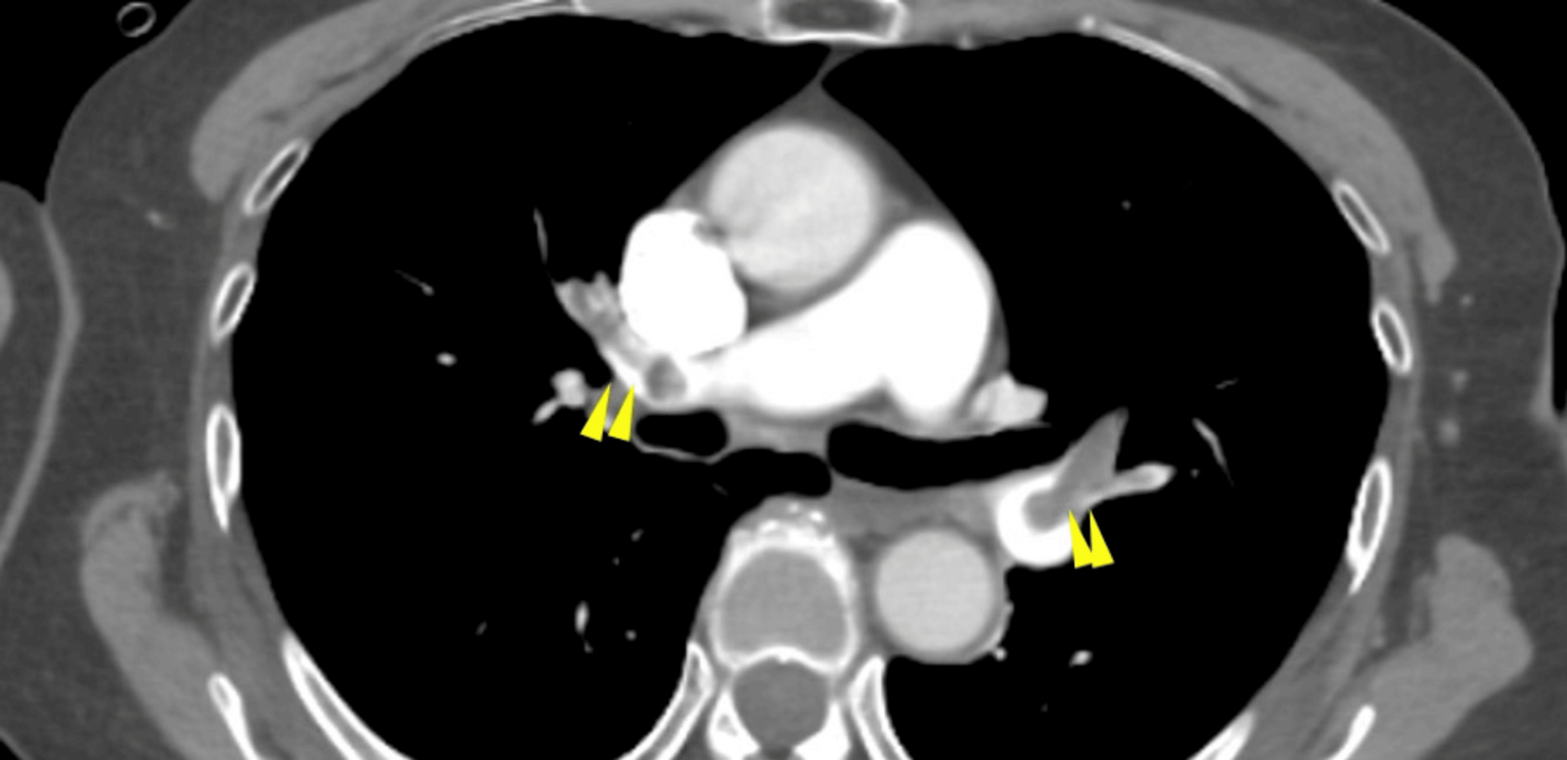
Contrast-enhanced CT revealed the presence of pulmonary artery thrombi (yellow arrowheads).
